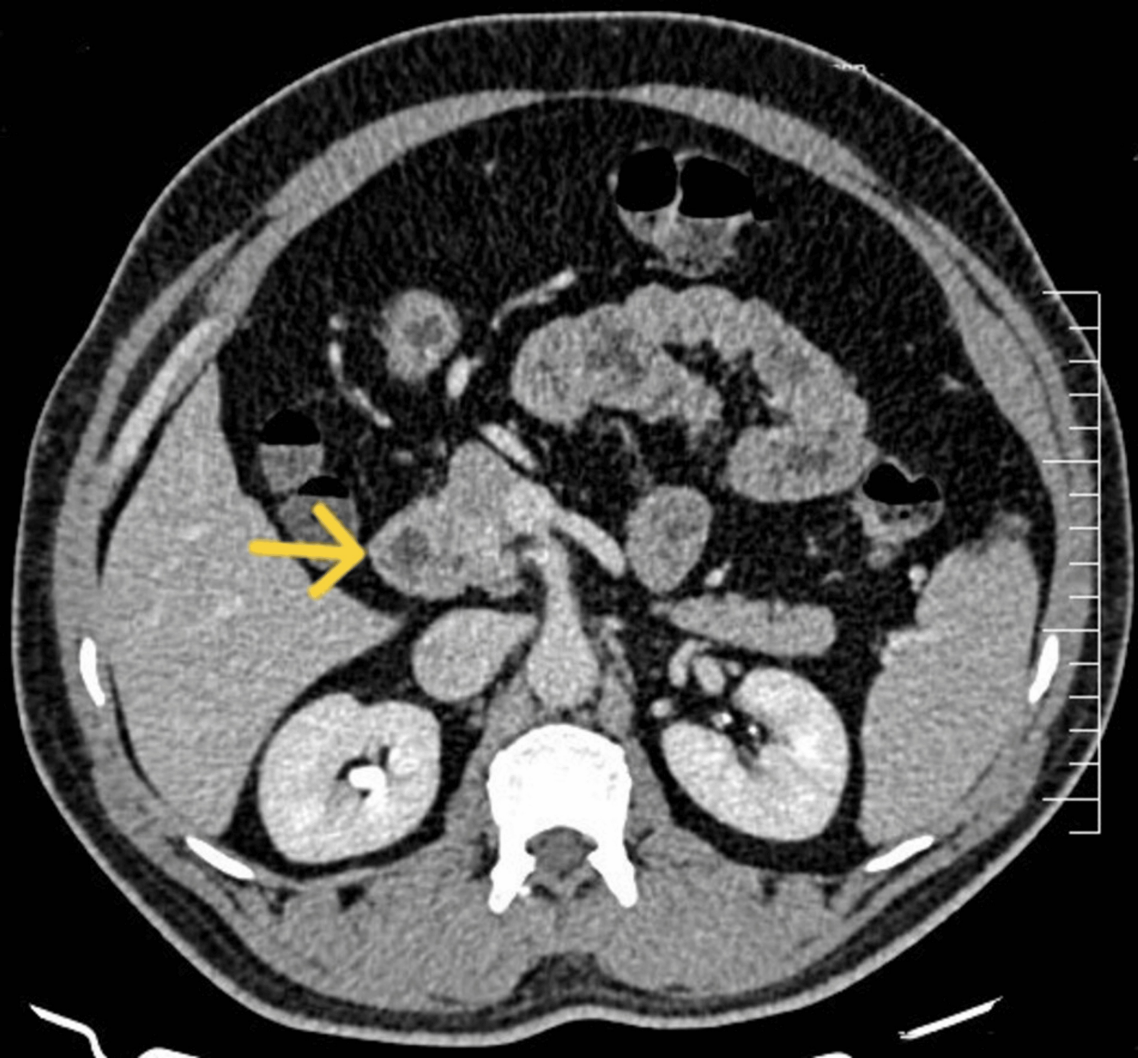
CECT of the abdomen demonstrating an ill-defined, heterogeneously hyperenhancing 21×15 mm lesion in the pancreatic head (arrow), consistent with insulinoma. CECT: contrast-enhanced computed tomography
